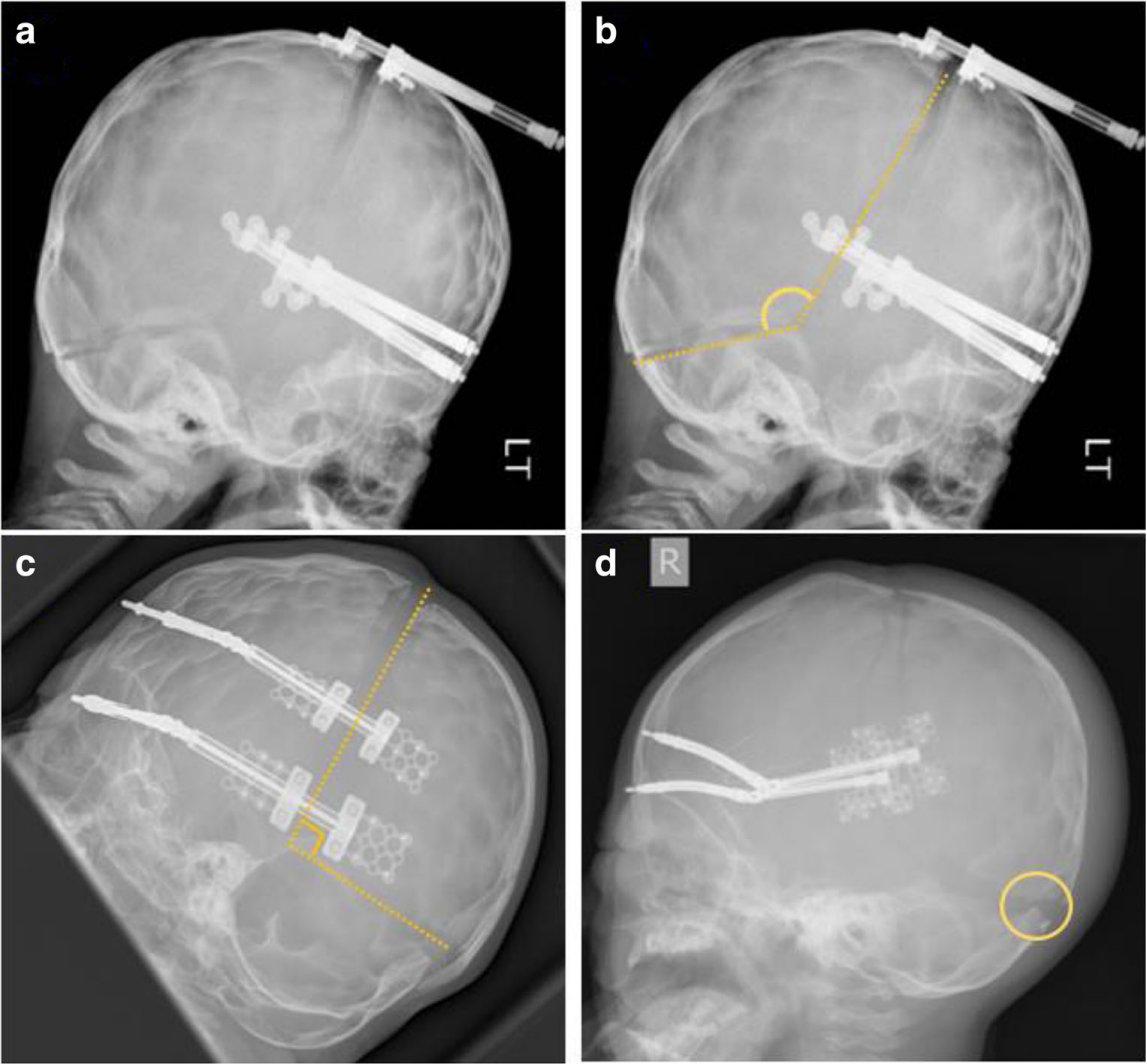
a A post-operative lateral plain film radiograph of a child, included in this study, having undergone infratentorial PCD. b A duplicate of panel a highlighting the osteotomies and the angle between them (yellow), confirming this child underwent infratentorial PCD. c A post-operative lateral plain film radiograph of a child, included in this study, having undergone supratentorial PCD—note the angle of the osteotomies (yellow) is approximately 90° and significantly different from that of panel b. d A post-operative lateral plain film radiograph of a child, included in this study, having undergone supratentorial PCD—note the torcular shelf viewable beneath the inferior osteotomy (yellow circle)
